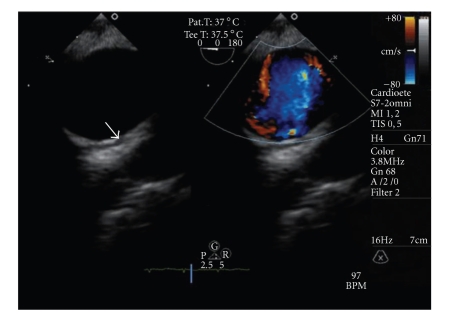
TEE image of the PDA, with sectioning plane at 0°, performed 4 weeks after surgery and before the discharge, showing the aortic conduit (*arrow)*; (left) with a normal colour doppler flow (right).
